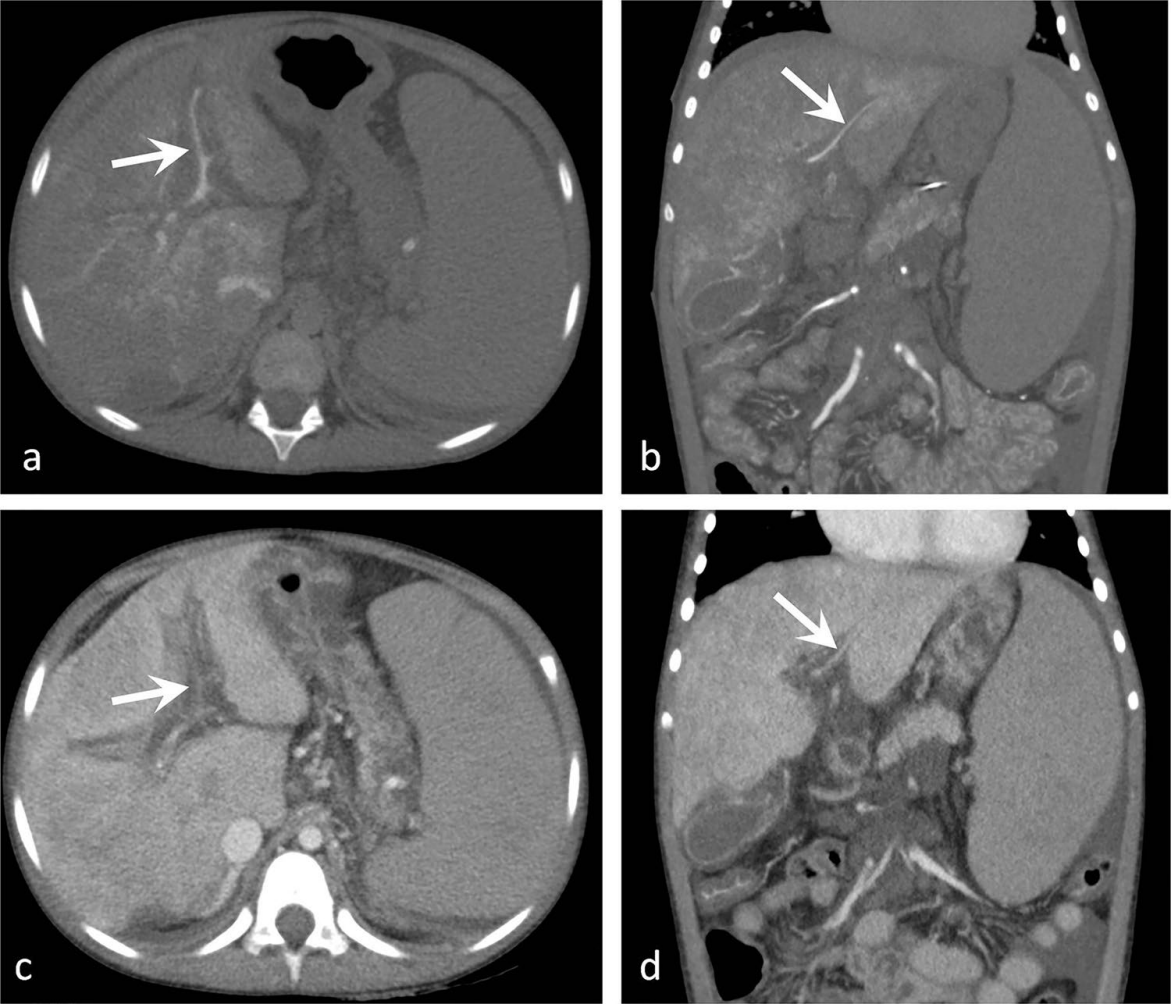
A 8-year-old female patient with PH. Coronal and axial images of CT-AP (a, b) and CE-CT (c, d). The left central and peripheral intrahepatic PV (arrows) were categorized "not reliably assessable" in CE-CT (c, d). CT-AP delineates the small, but clearly patent left intrahepatic PV.
